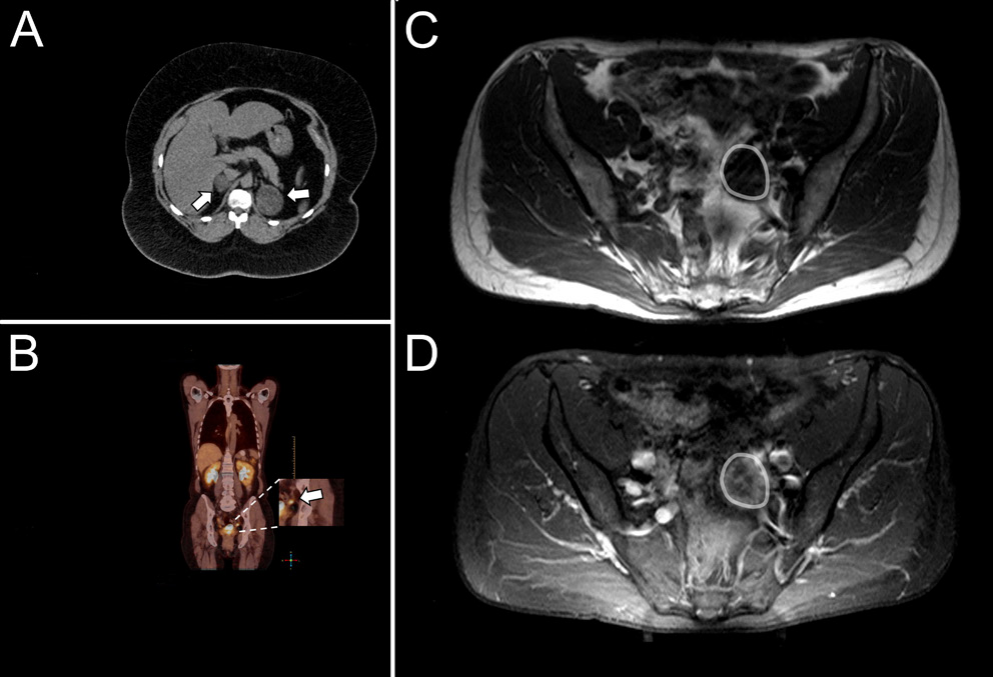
Computed tomographic scan showing bilateral macronodular adrenal hyperplasia (Patient 1, A); PET-CT revealing a left-sided ovarian mass (Patient 2, B); MRI scan revealing a cystic tumor on the left ovary (C) with peripheral contrast media enhancement (Patient 3, D).
